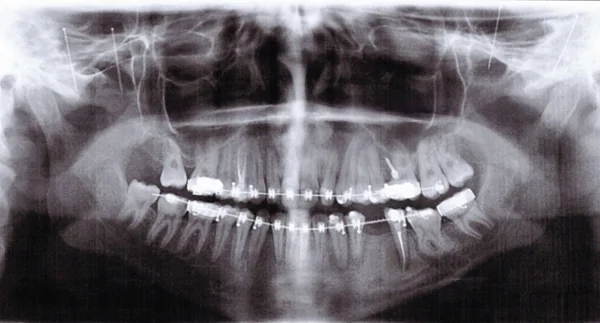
Sequential panoramic radiographs.
